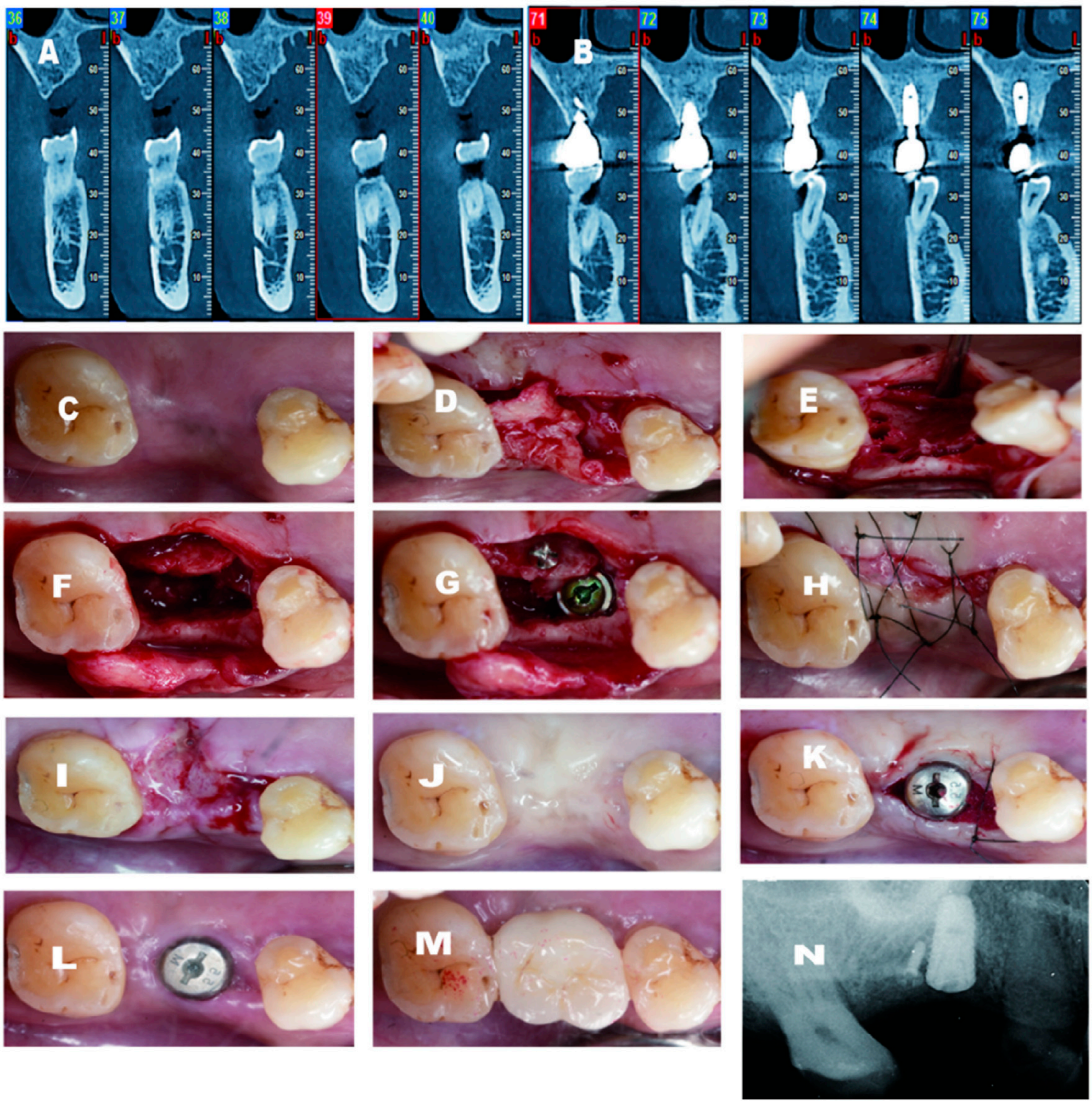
(A) Pre-operative CBCT demostrates large palatal defect; (B) 2 years follow-up CBCT shows excellent gain on the palatal aspect; (C) Pre-operative-occlusal view; (D) Palatal flap elevated; (E) C-shape scored; (F)Ridge split conducted; (F) Implant installed and titanium screw secured the migrated palatal bone; (H) Suture completed; (1)2 weeks after surgery; (J) 4 months after surgery; (K) Second stage surgery and healing abutment installed with secondary healing intension mesially; (L,M) 2 weeks after second stage and final crown delivery; (N) Periapical X-ray before second stage.
